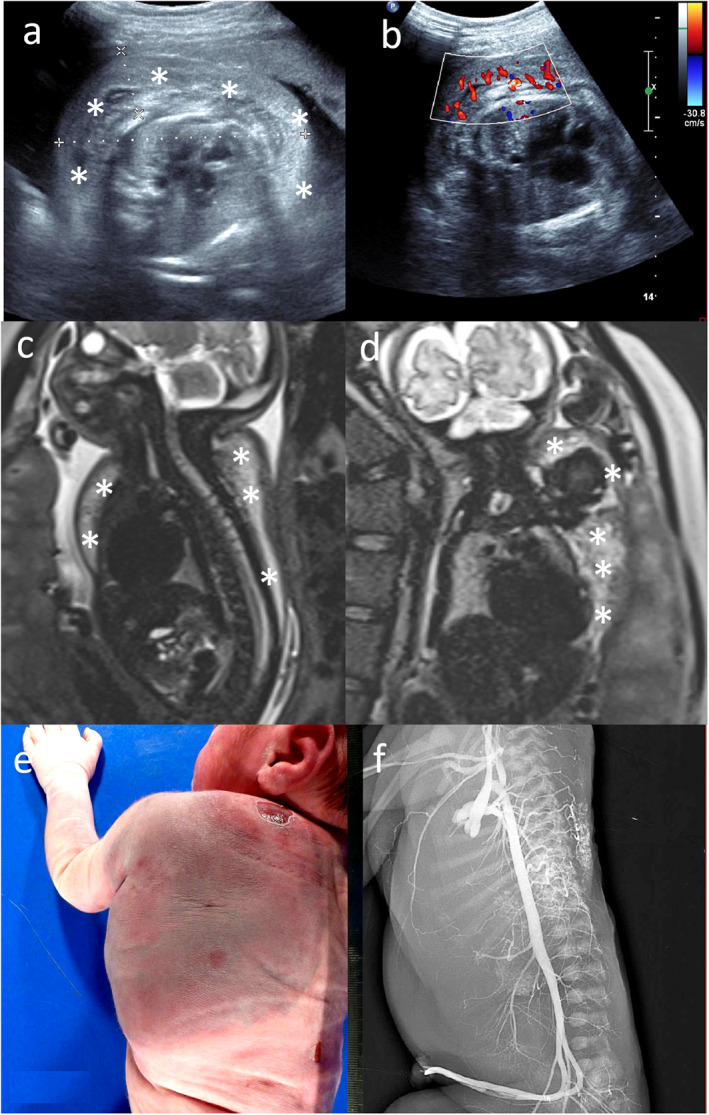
(a–f, Case 2). Axial Doppler‐ultrasound images at 35 weeks' gestation show an echogenic, markedly hypervascular infiltrative mass of the left thoracic wall, involving both subcutaneous tissues and the intercostal musculature (a, b). These findings correspond to the T2‐hyperintense infiltrative lesion seen on midsagittal and coronal fetal MRI, which better delineates the extent of deep‐tissue involvement (c, d). Given the severity of the lesion, the parents elected termination of pregnancy. Macroscopic examination confirmed the prenatal imaging features and demonstrated the characteristic violaceous skin discoloration of KHE (e). Postmortem angiography via the umbilical artery revealed arterial supply from multiple intercostal branches arising from the thoracic aorta (f).
